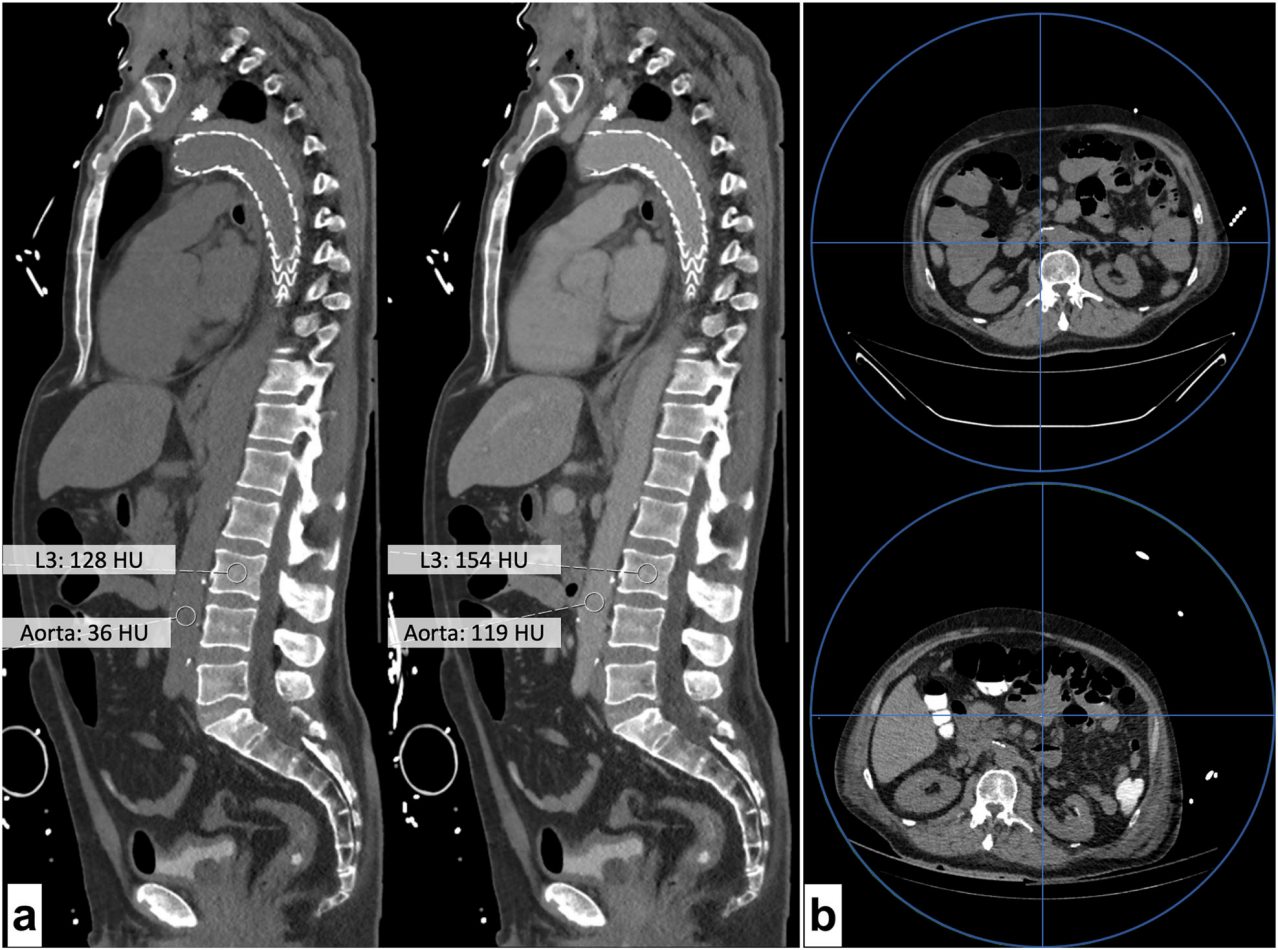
a Sagittal reformations of two thoracolumbar CT scans (left, unenhanced; right, 90 s after intravenous contrast media administration). Scans were obtained for search of an endoleak of the aortic prothesis (arrowhead). Annotations show HU of the abdominal aorta and the L3 vertebral body. Measured bone mineral density was 96 mg CaHA/cm^3^ in the unenhanced scan and 98 mg CaHA/cm^3^ in portal-venous phase. b Axial reformations of two unenhanced CT scans of a single patient obtained 20 days apart on the same scanner (Philips IQon). Images show cross-sections at the L3 level. Blue circles represent the maximum field of view. Blue lines intersect at the scanner center. The substantial difference in patient placement and distance between the lumbar spine and the scanner center between scans is evident and may explain substantial differences in bone mineral density measurements (top, 207.7 mg CaHA/cm^3^; bottom, 227.2 mg CaHA/cm^3^). Of note, the bottom scan was obtained following oral contrast administration
